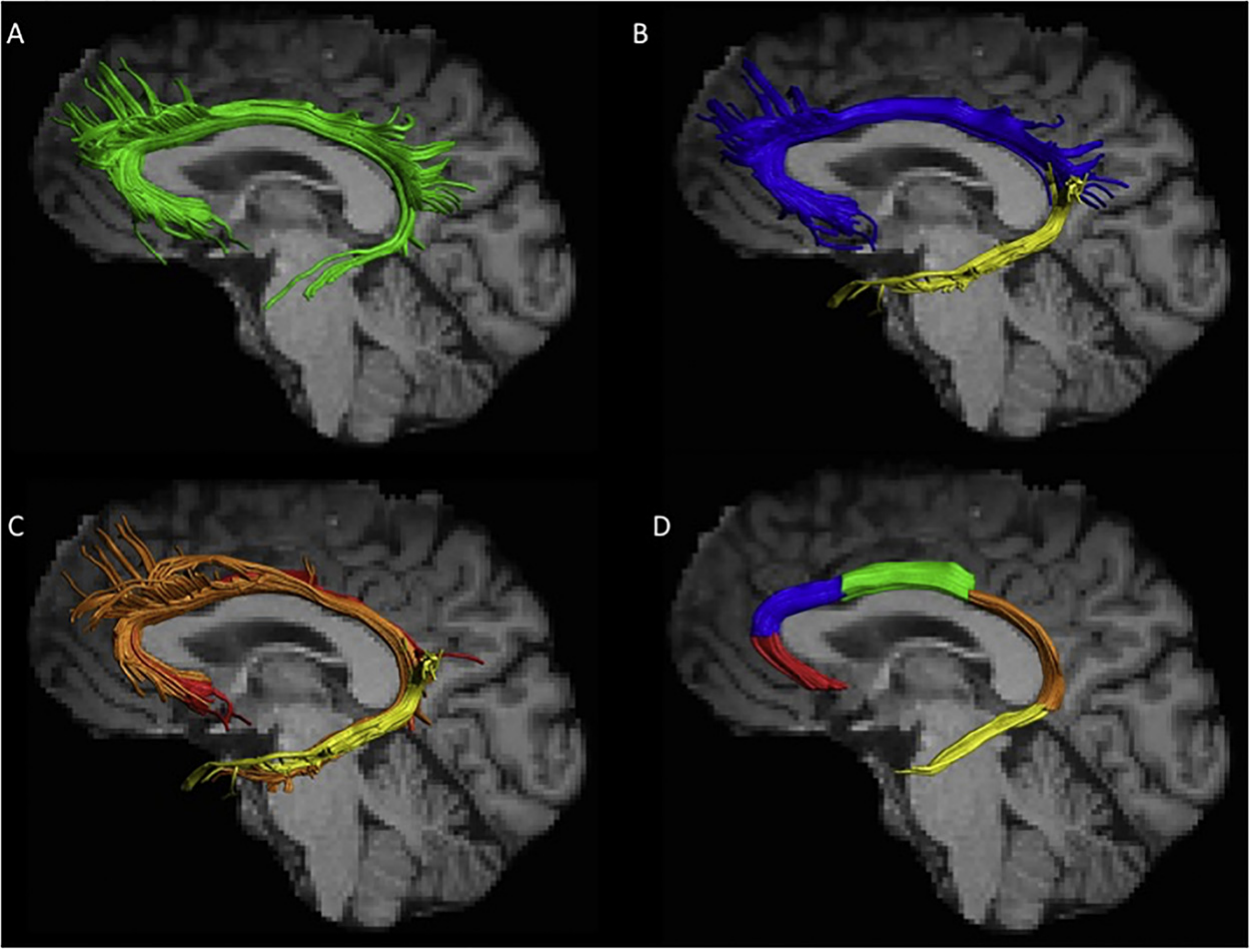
Cingulum bundle reconstructions based on diffusion MRI tractography. Images show the left cingulum for one healthy individual displayed on a T_1_-weighted image. A) The cingulum reconstructed as a single, continuous bundle (green), B) Dorsal (blue) and ventral (yellow) cingulum subdivisions (e.g., Budisavljevic et al., 2015). C) Subgenual (red), retrosplenial (orange), and parahippocampal (yellow) cingulum subdivisions (Jones et al., 2013). D) Proposed subdivisions along the longitudinal axis, with subgenual (red), anterior cingulate (blue), midcingulate (green), retrosplenial (orange), and parahippocampal (yellow) portions. Note that additional streamlines, e.g., in frontal and parietal areas, have been removed (For interpretation of the references to colour in this figure legend, the reader is referred to the web version of this article).
